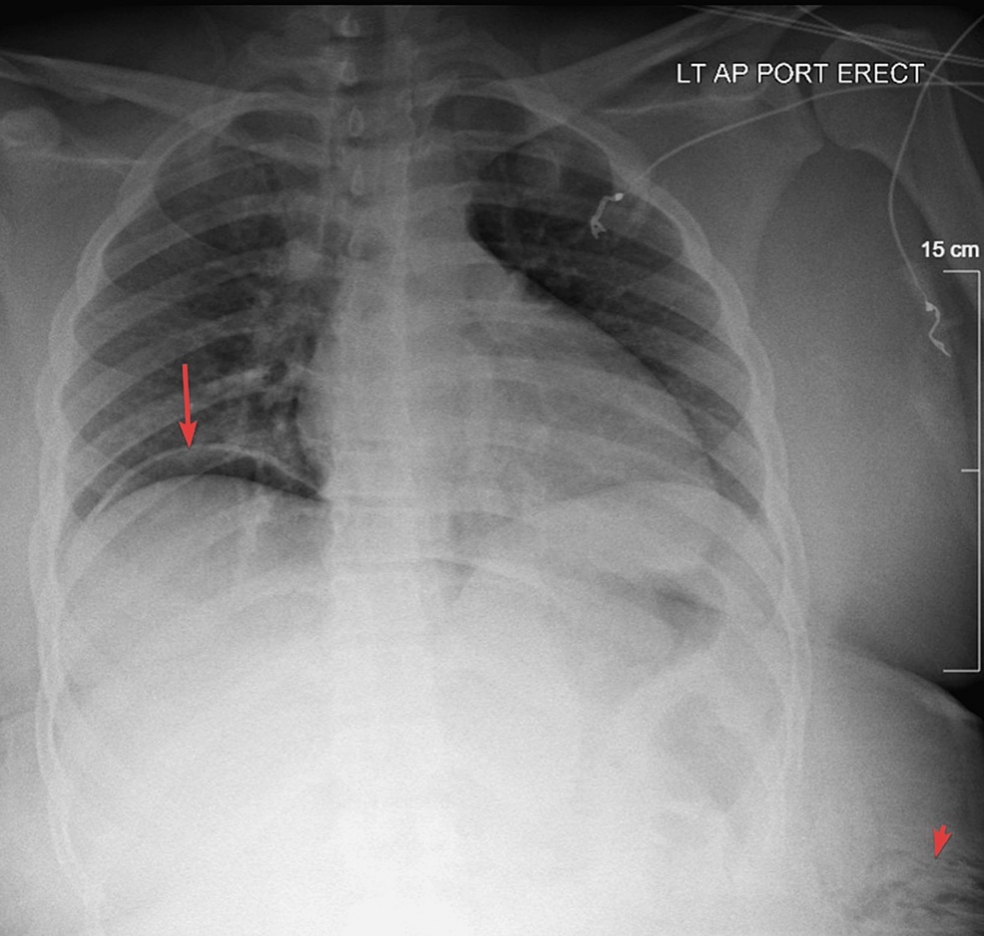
Upright chest X-ray that included the portion of the abdomen. Free intraabdominal air under the right diaphragm is marked with a long arrow, and subcutaneous air in the left middle flank area is marked with a short arrow.
